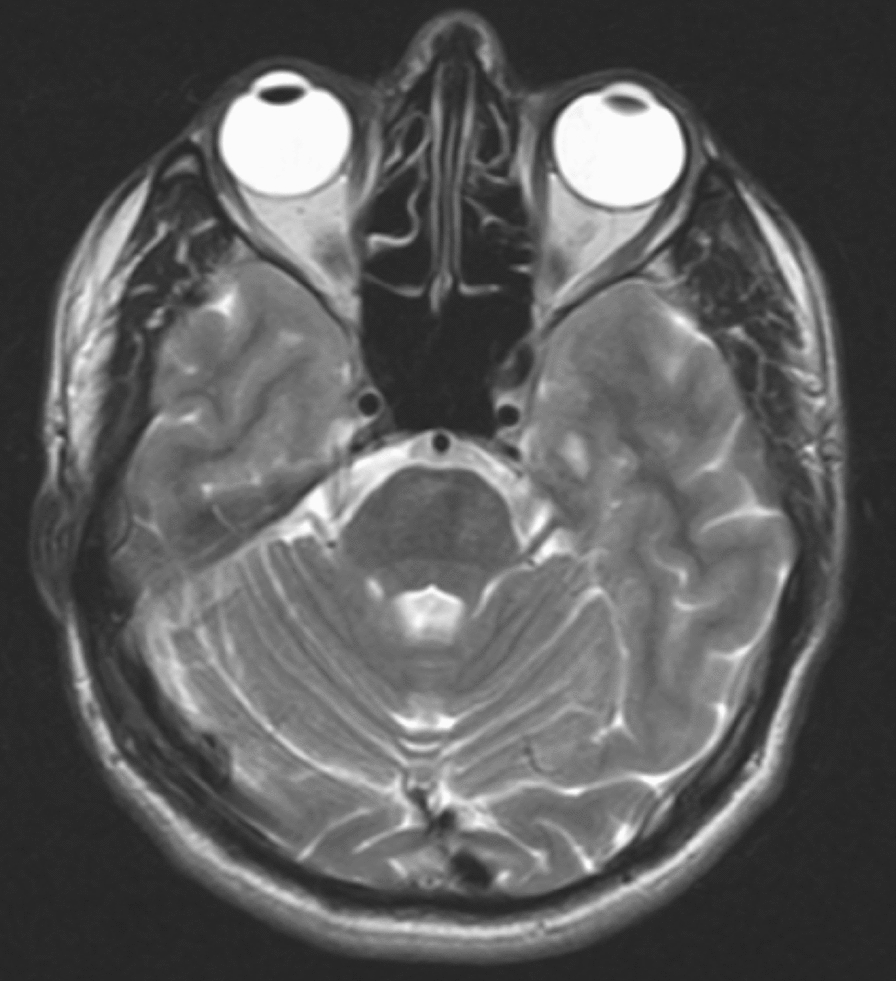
Axial T2-weighted brain MRI showed a clear course of left facial nerve
